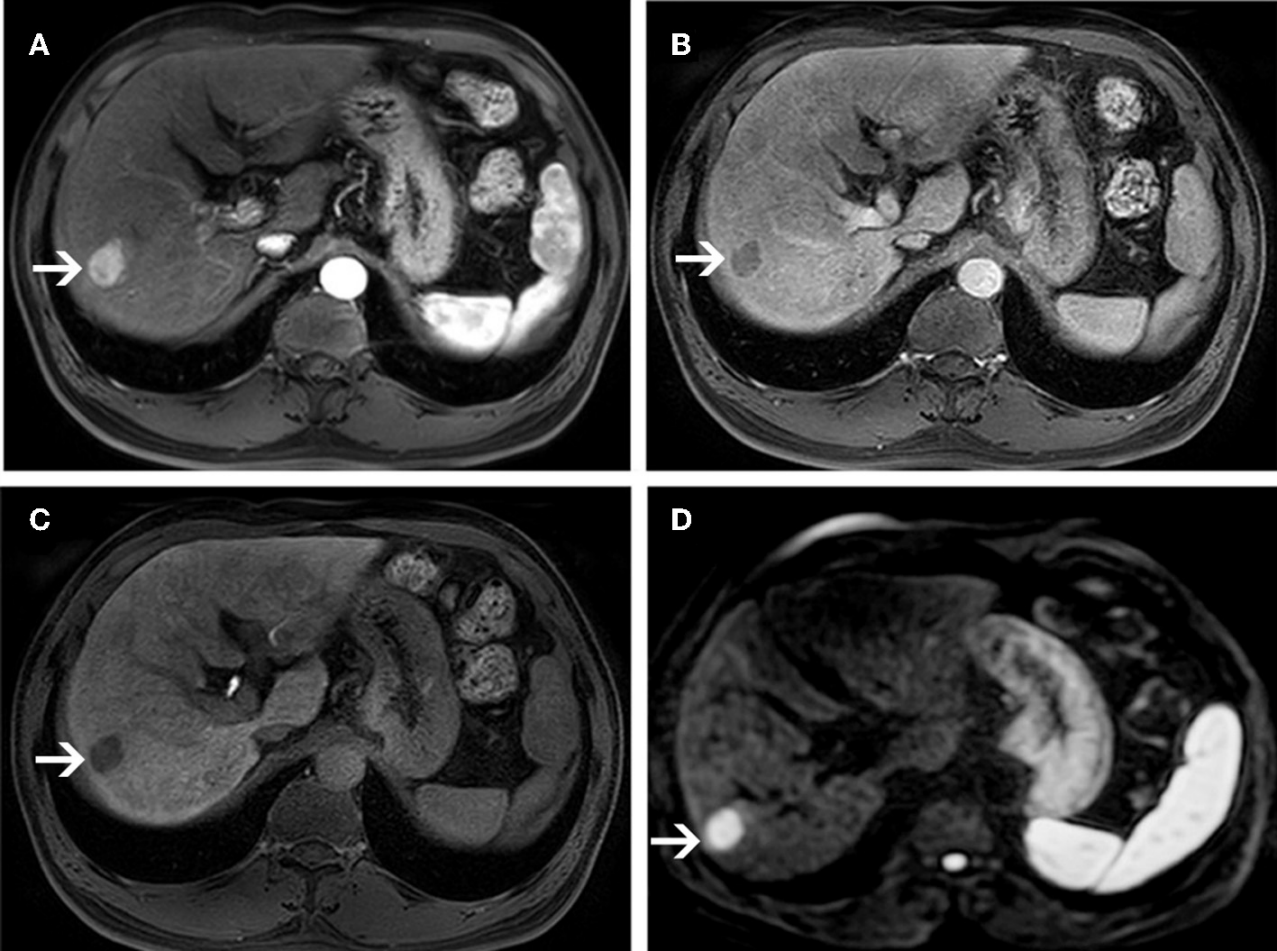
MR images of a 58-year-old man with a pathologically proven HCC (white arrows) and a history of hepatitis B virus infection. An arterial-phase image (A) shows an enhancing nodule in segment VI of the liver. An equilibrium phase MR image (B) shows a nodule demonstrating washout of contrast material, and showing capsular appearance. At the hepatobiliary phase (C), the lesion is hypointensity compared to the surrounding liver parenchyma. On diffusion-weighted image (D), the lesion is hyperintensity compared to the surrounding liver parenchyma.
